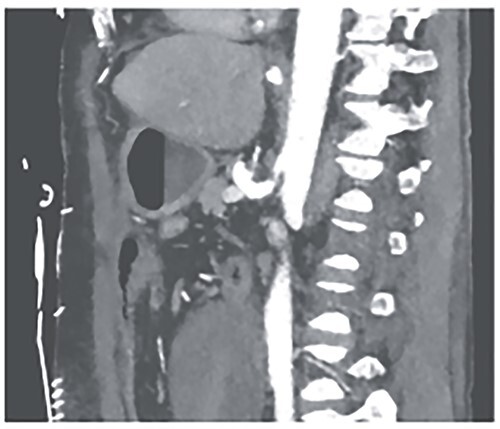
CTA showing a relevant stenosis of the celiac trunk.
